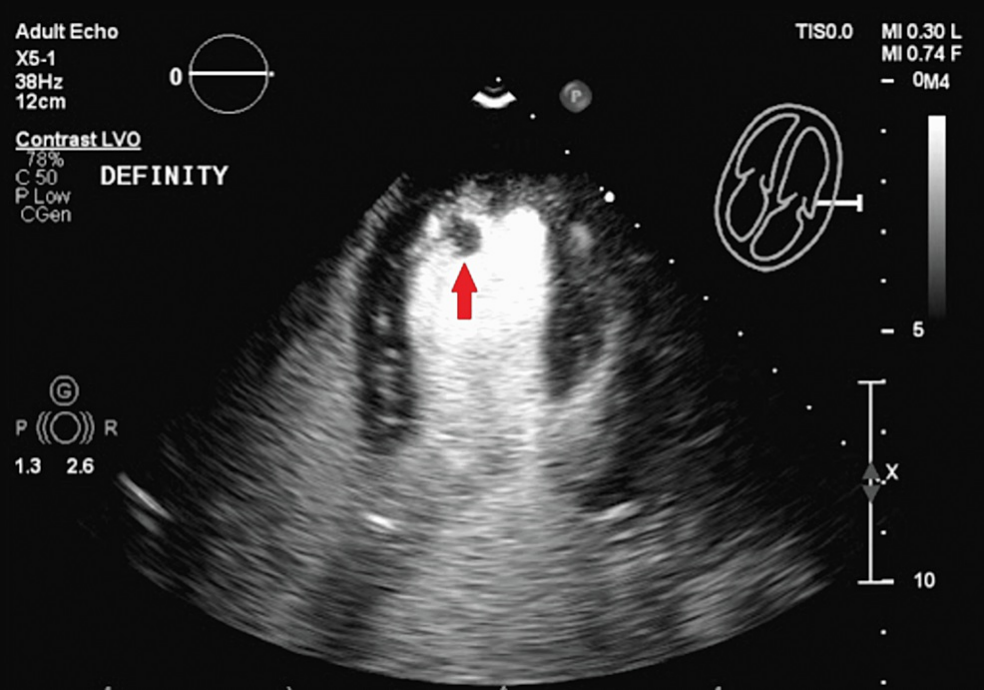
Transthoracic echocardiogram with a contrast agent, demonstrating left ventricular apical thrombus (red arrow)
